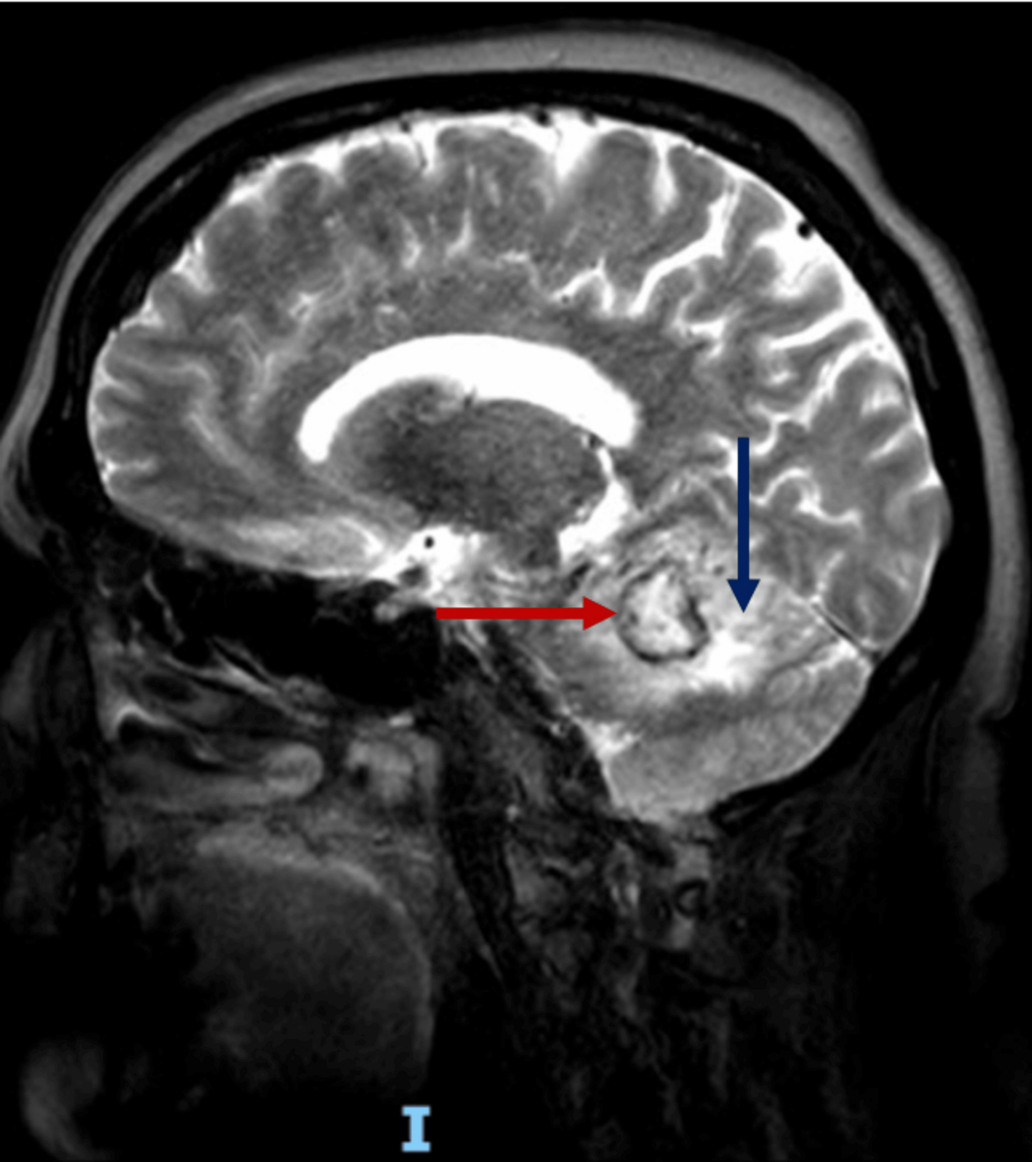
T2-weighted brain magnetic resonance image Ring-enhancing lesion (red arrow) in the right cerebellar hemisphere with edema extending toward the right fifth cranial nerve and the ipsilateral recess of the fourth ventricle (blue arrow)
